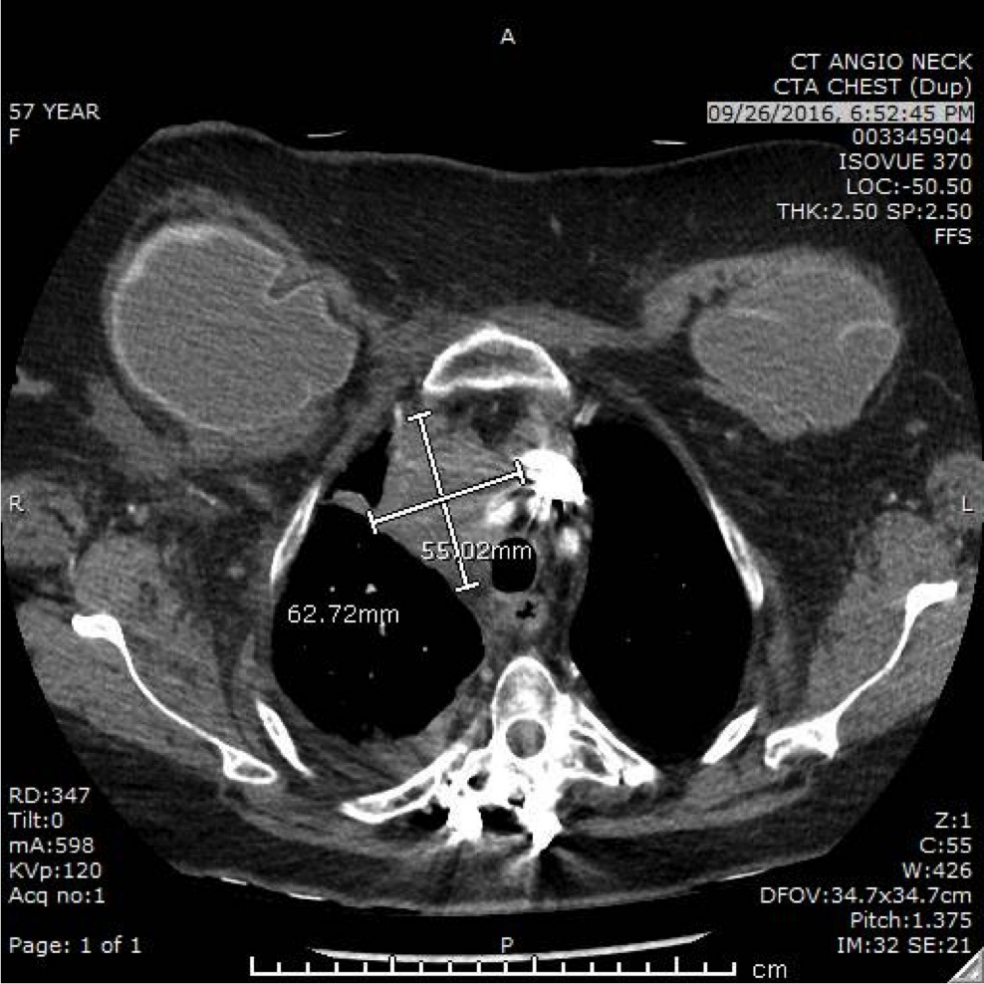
Computed tomography angiography (CTA) scan of the chest before initiation of Olaparib therapy
